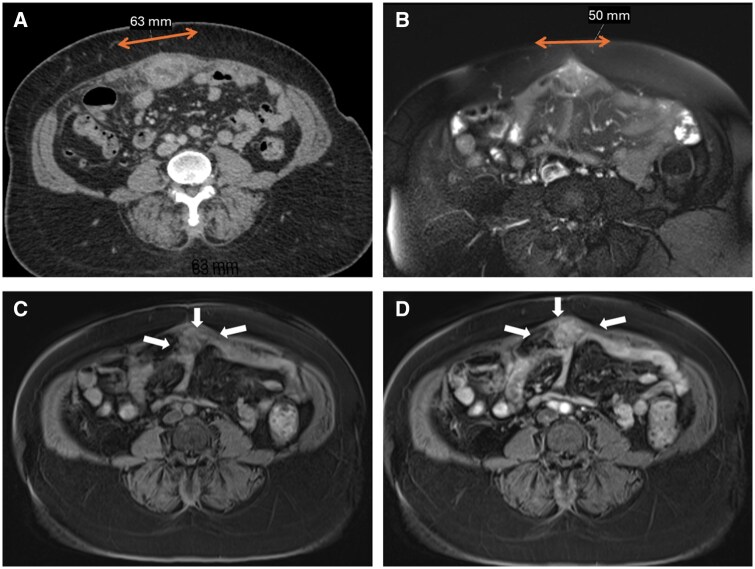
Case 3. Axial (A) CT with PV contrast image showing 63-mm anterior abdominal wall inflammatory mass, followed by an axial T2 fat saturated image (B) from the MRI acquisition performed 1 month following the CT, showing reduction in size. The mass was still displaying enhancing components on MRI, as seen on T1 fat saturated pre-gad (C) and post-gad (D) imaging.
